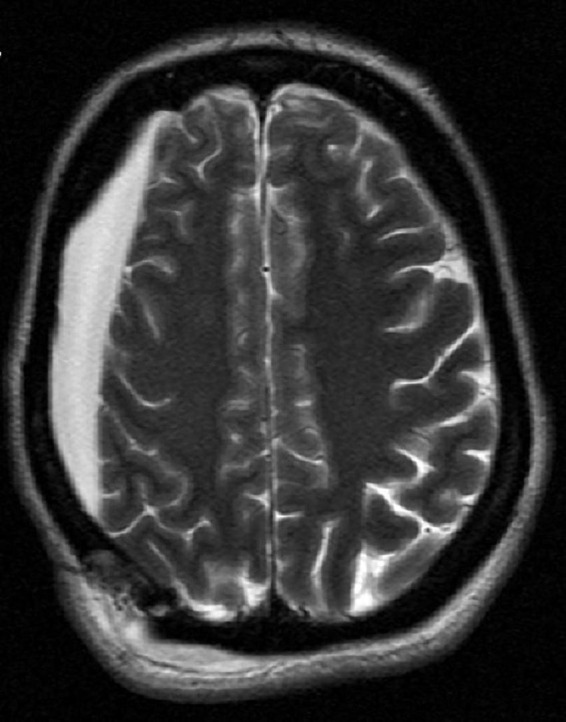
Magnetic resonance imaging showing patient’s right hygroma prior to initiation of electroconvulsive therapy (ECT).
